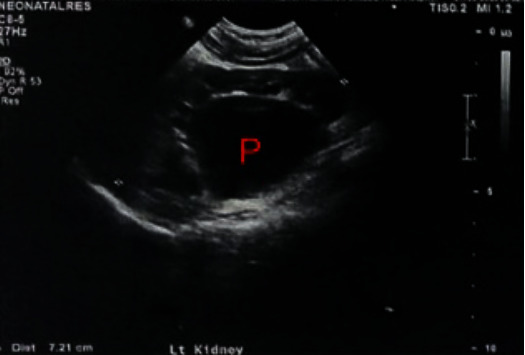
Ultrasound showing severe left PUJO at presentation.
